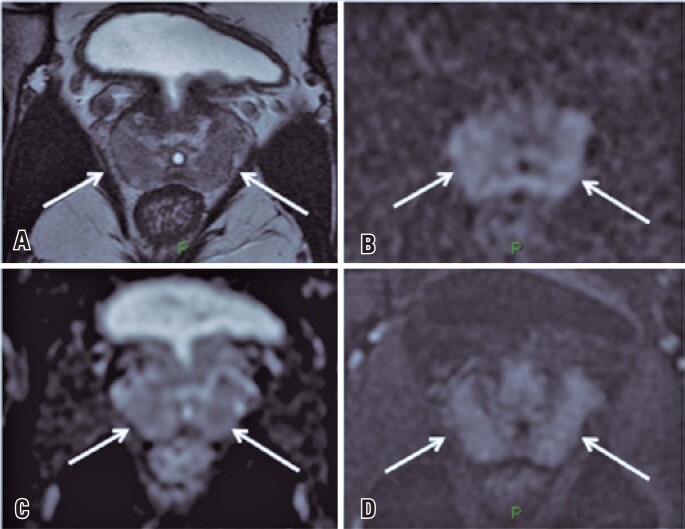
Prostate magnetic resonance imaging of a 39-year-old man with suspected acute prostatitis. The imaging study shows aspects that resemble those seen in figure 1 (in an asymptomatic patient): diffuse hypointensity on T2W (arrows in A), with areas of diffusion restriction seen on DWI and ADC map (arrows in B and C) and early diffuse contrast enhancement (arrows in D)
